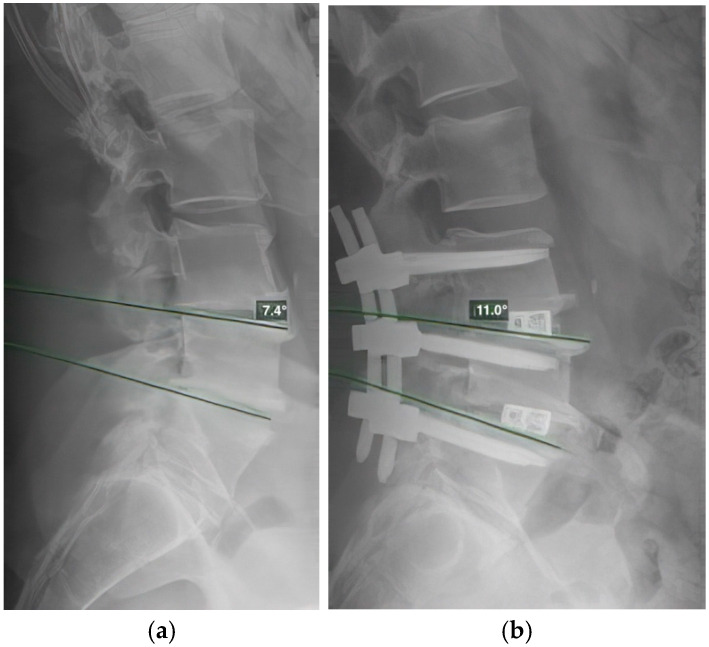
Measurement of segmental lordosis, defined as the angle between the tangent lines and the superior endplates of two adjacent vertebrae. (a): Lateral radiograph of the lumbar spine showing preoperative segmental lordosis and the measured segmental lordosis. (b): Lateral radiograph of the lumbar spine 6 months postoperatively, following spondylodesis of L3–L5 with 10° TLIF cages, showing the measured segmental lordosis of the L4–L5 segment.
